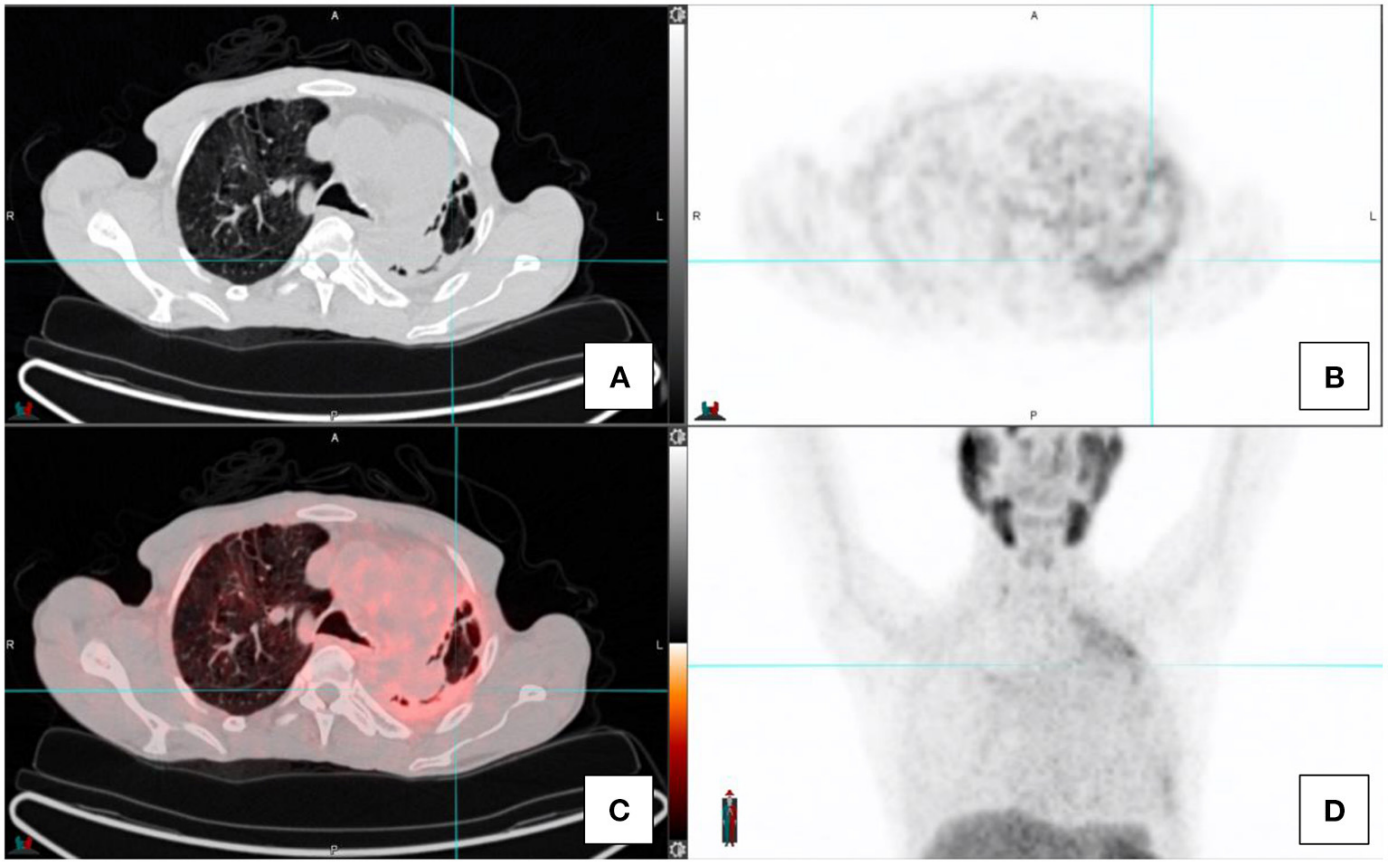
Transaxial images in a prostate cancer patient with concomitant fibro-cavitatory pulmonary tuberculosis. The patient was recently started on treatment for tuberculosis. CT-image (A), PET image (B), fused PET/CT image (C), and the maximal intensity projection image (D).
